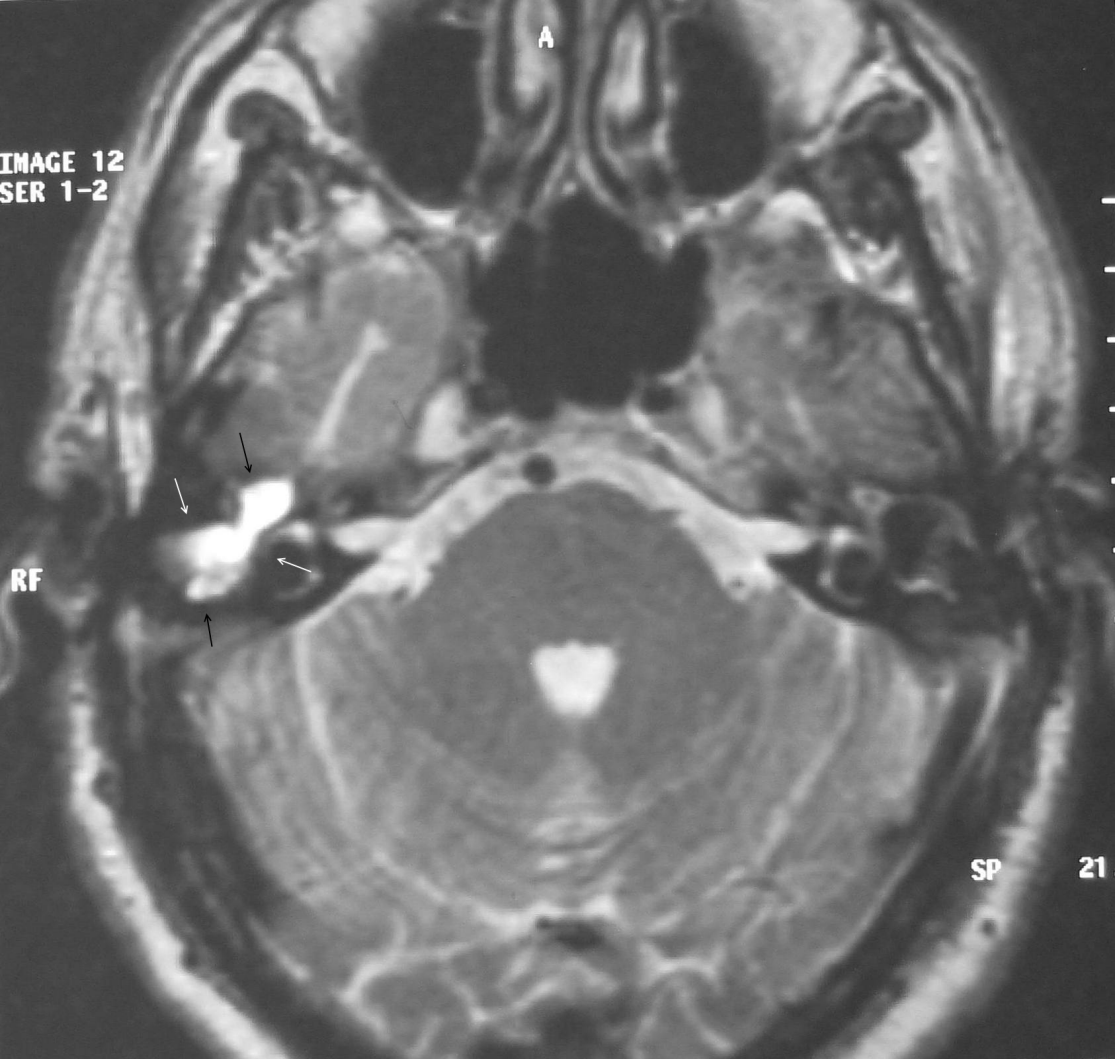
T2-weighted temporal bone MRI showing a homogenous mass with increased signal intensity relatively to the brain. Arrows indicate the mass.
